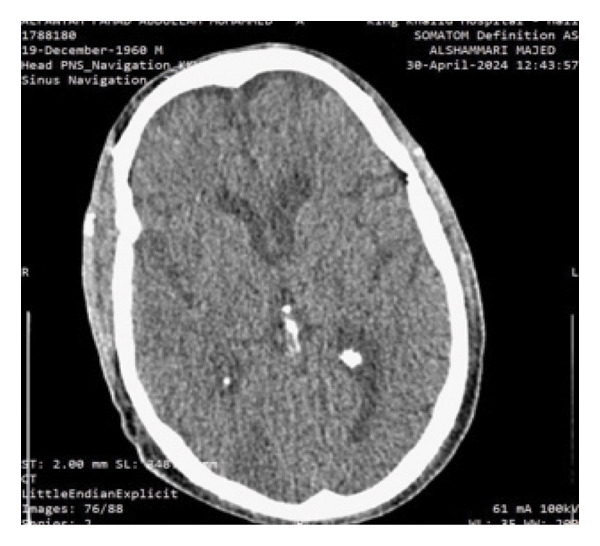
Axial MRI/CT showing cavernous sinus invasion and multiple acute cerebral infarcts in the right hemisphere.
